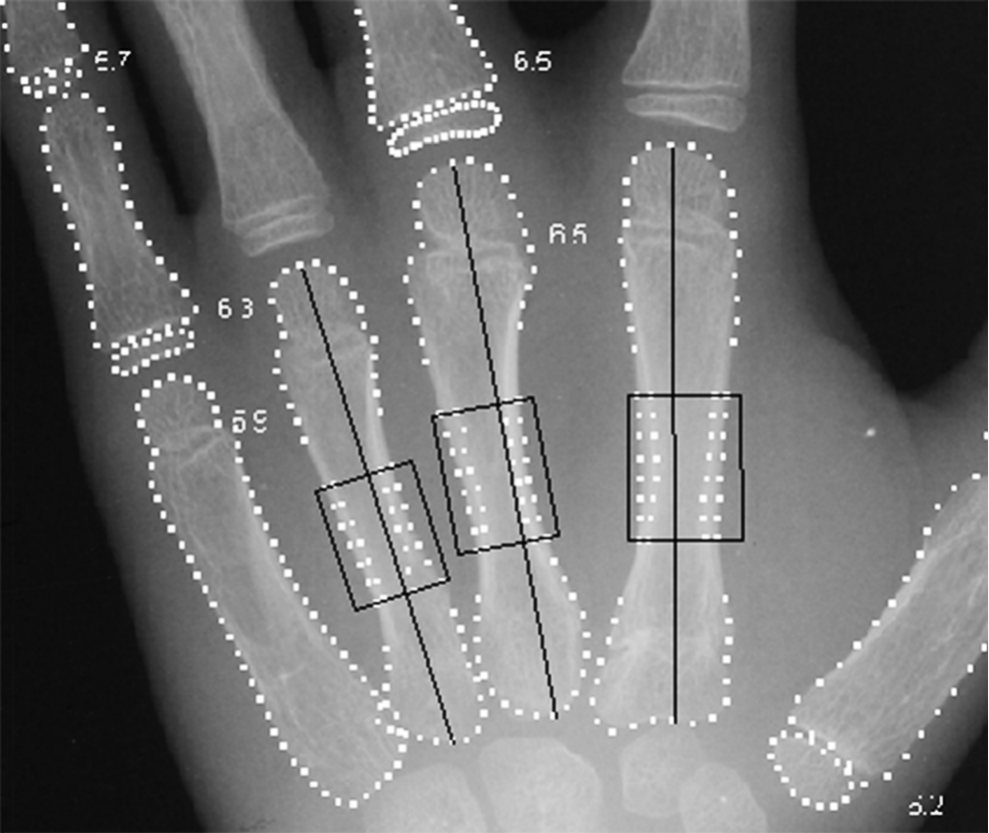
Bone age. Hand radiograph of a 6-year-old girl shows the bone borders used for calculating bone age (*dotted white lines* around the individual bones, or ossification centres) and paediatric bone index (*black boxes* in metacarpals II through IV), as outlined by BoneXpert. The small numbers represent the given bone age for the individual bones used in calculating bone age
